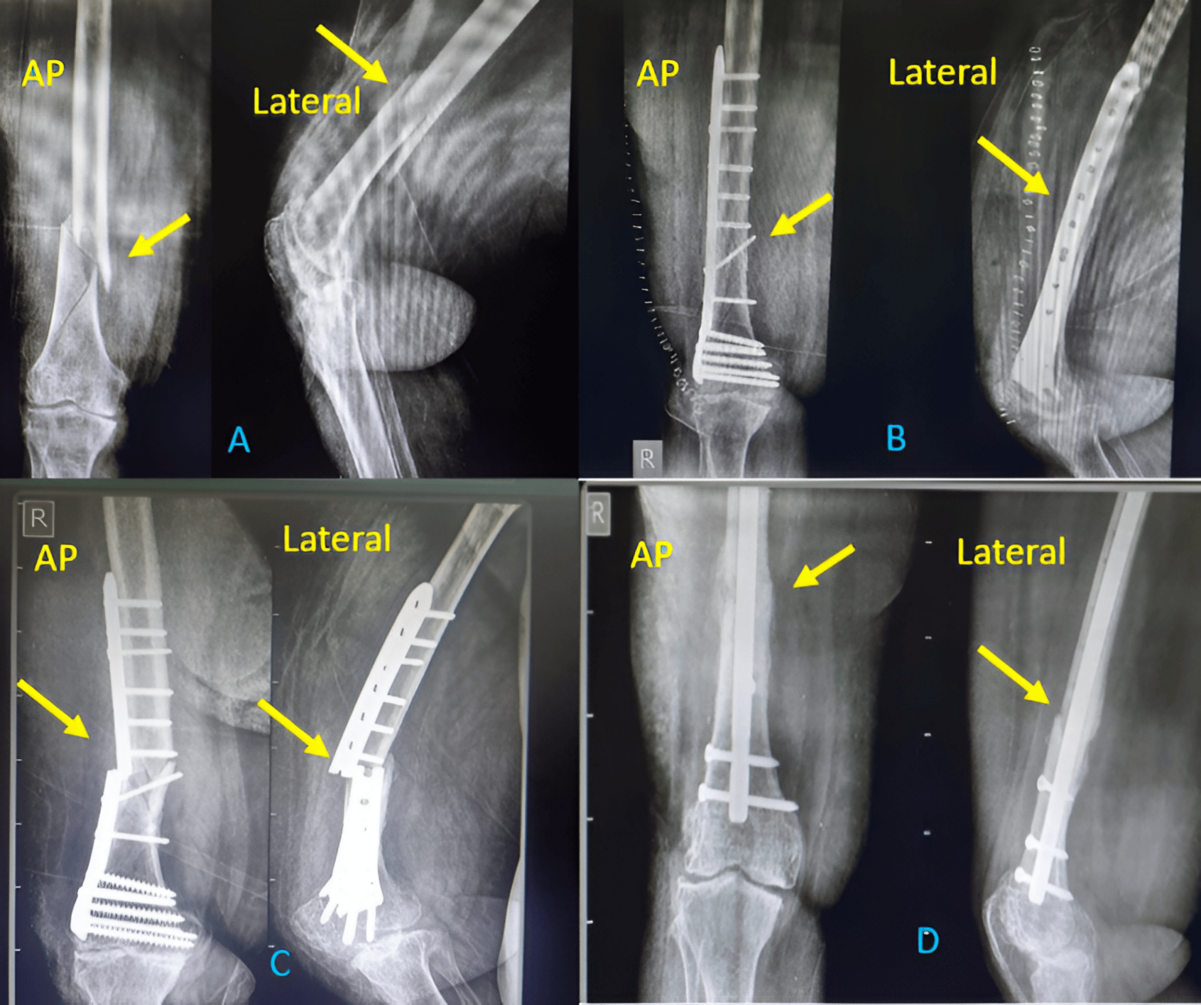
Poor results obtained with DFLCP (A) AP and lateral X-rays after trauma (arrow showing fracture), (B) AP and lateral X-rays after surgery (arrow showing fracture), (C) AP and lateral X-rays on follow-up (arrow showing broken plate), and (D) AP and lateral X-rays after revision surgery (arrow showing united fracture) DFLCP: Distal Femur Locking Compression Plate
